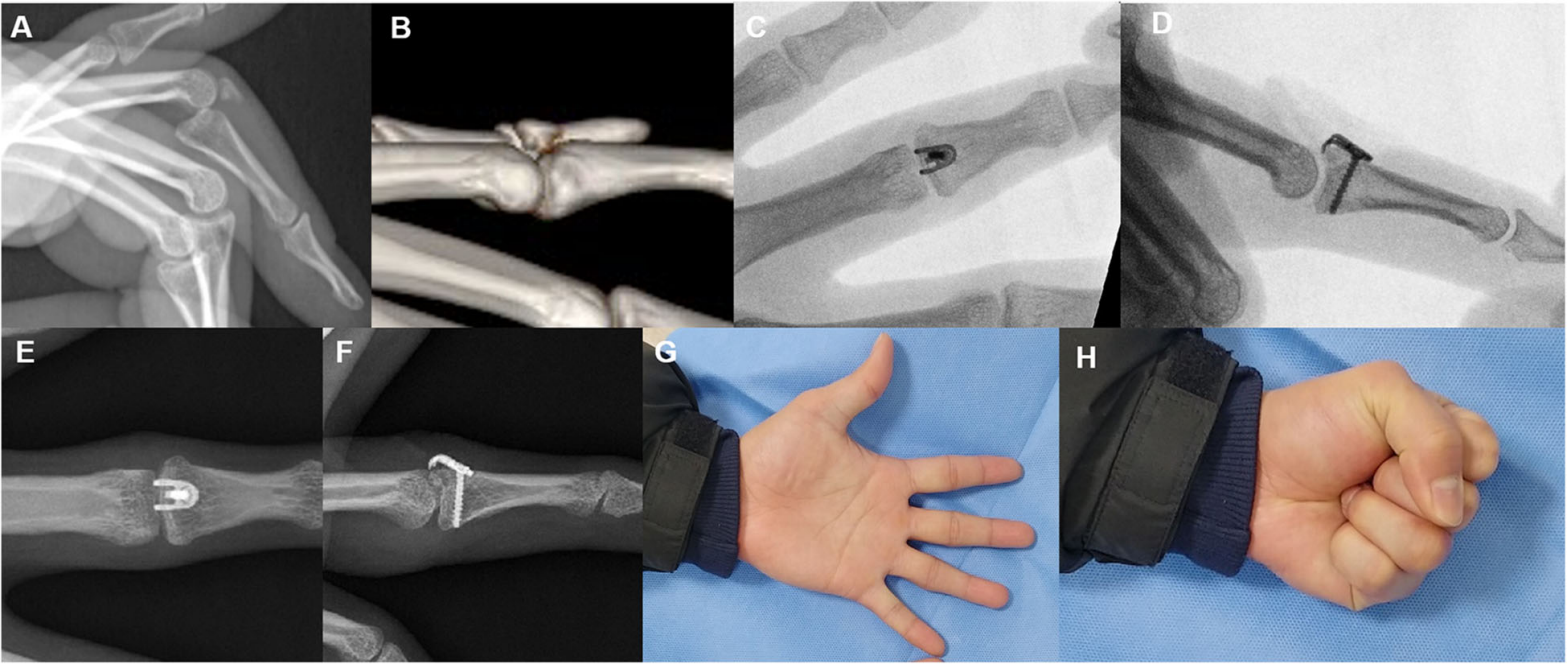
Central slip avulsion fracture with volar subluxation of PIP joint (a, b). The mini-hook plate captured the dorsal avulsed fragment, and the joint was reduced (c, d). Plain radiography 3 years post-operation revealing that osseous fragment was well united and that there was no metal failure or joint subluxation (e, f). The total range of PIP joint motion was 95%, and the pinching strength was 90% compared to the values of the same contralateral joint (g, h)
